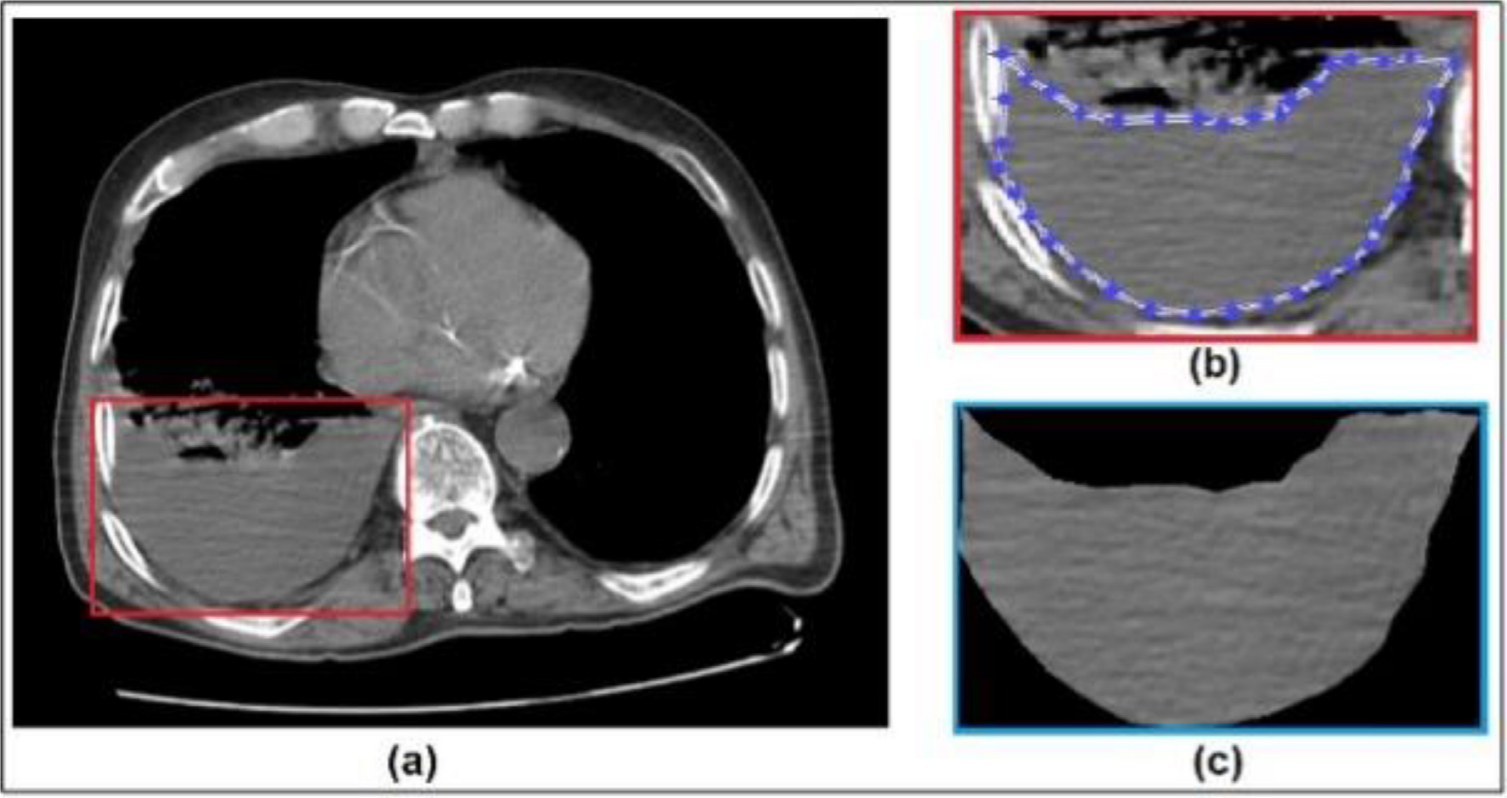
Full size Chest CT image with pleural effusion (1a), region of interest for pleural effusion (1b), extracted feature of pleural effusion (1c).
